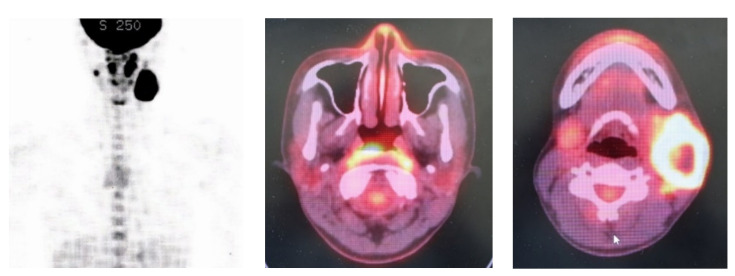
PET-CT could show accumulations in both the left neck lymph and nasopharynx, but nowhere else in the body.
